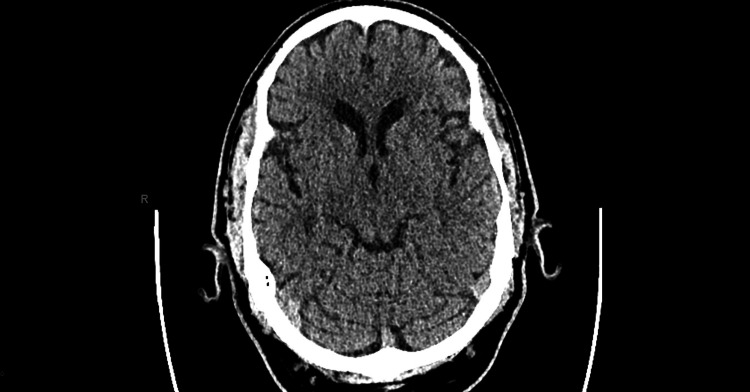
CT scan of the brain; no acute intracranial abnormality
